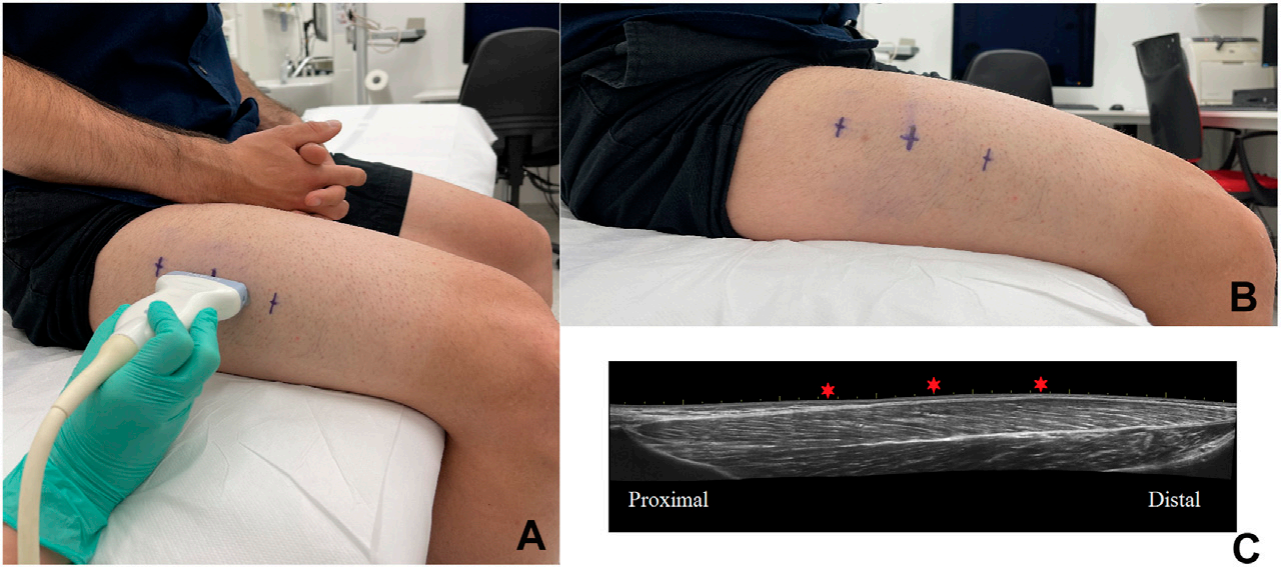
Ultrasonography measurement on vastus lateralis and the points of measurements on the skin (A,B). Sagittal plane panoramic ultrasound image of right leg’s m. quadriceps femoris—vastus lateralis of same individual; the red stars sign the measurement points (C).
